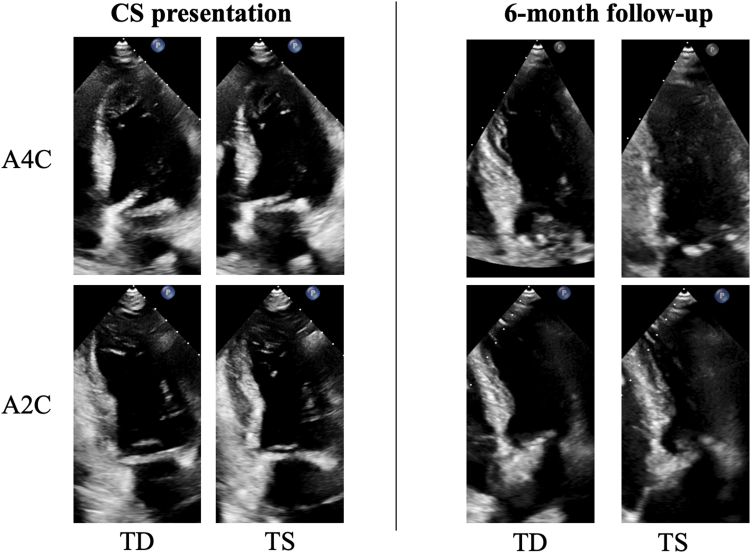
Echocardiographic demonstration of reversible left ventricular dysfunction. Initial transthoracic echocardiography in A4C and A2C views showing severe left ventricular dysfunction with ejection fraction of 10%-15%. Follow-up transthoracic echocardiography in A4C view showing complete recovery with ejection fraction of 65%-70% and normal prosthetic valve function. A2C, apical 2-chamber view; A4C, apical 4-chamber view; CS, cardiogenic shock; TD, telediastolic; TS, telesystolic.
